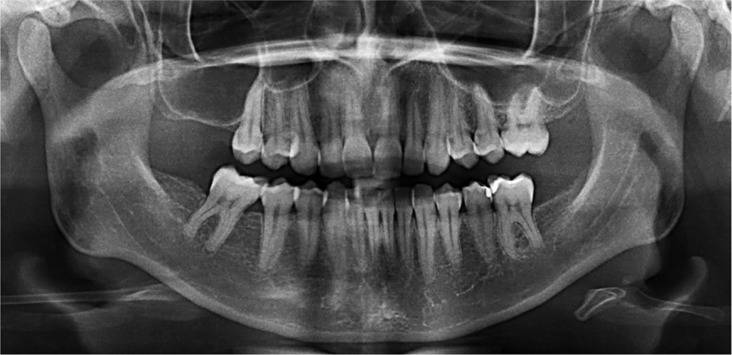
Panoramic radiography exhibiting absence of bone involvement.
